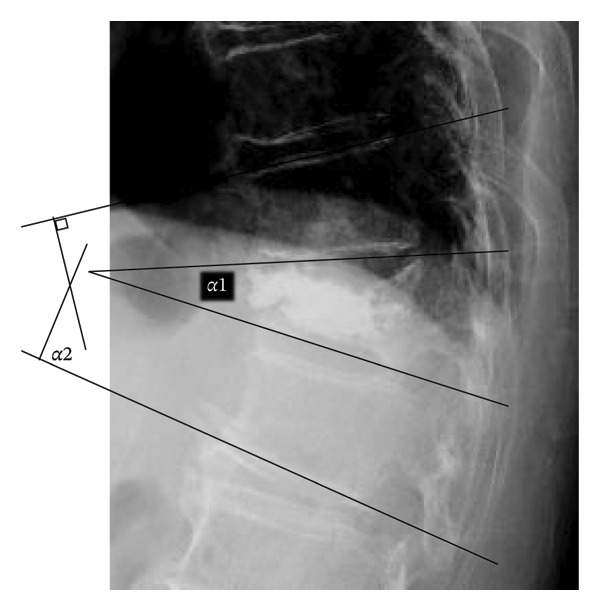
Parameters for sagittal alignment include the lateral wedge angle (α1) and the Cobb angle between proximal and distal adjacent vertebrae (α2: regional kyphosis angle) on lateral radiographs. These angular parameters were used to assess local sagittal alignment and postural instability at the fractured segment.
